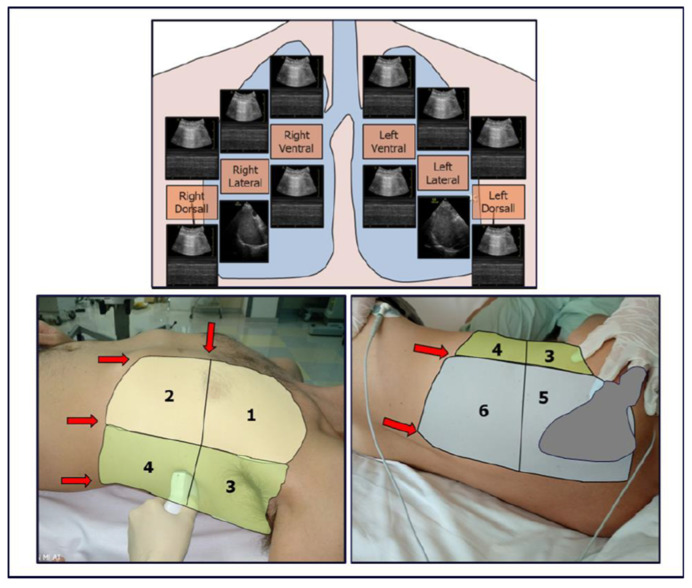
Thoracic zones (1–2 anterior, 3–4 lateral, 5–6 dorsal) are used to calculate the Classic Lung Ultrasound Score. A second operator is required to assist in assessing the dorsal zones in a supine patient undergoing mechanical ventilation. The presence of ribs and the scapula limits access to the pleural surface.
